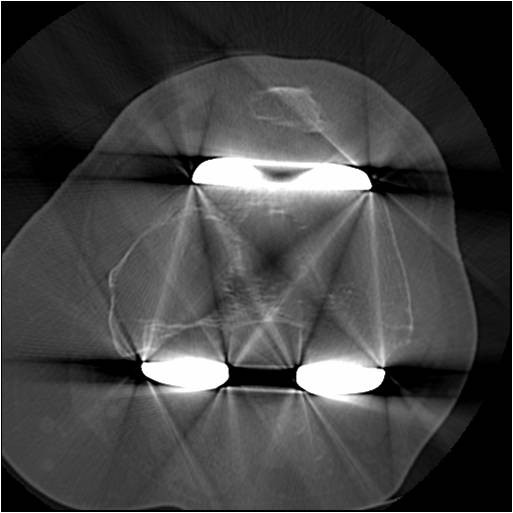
Axial CT of Femur demonstrating large lytic area in the medial femoral condyle.
